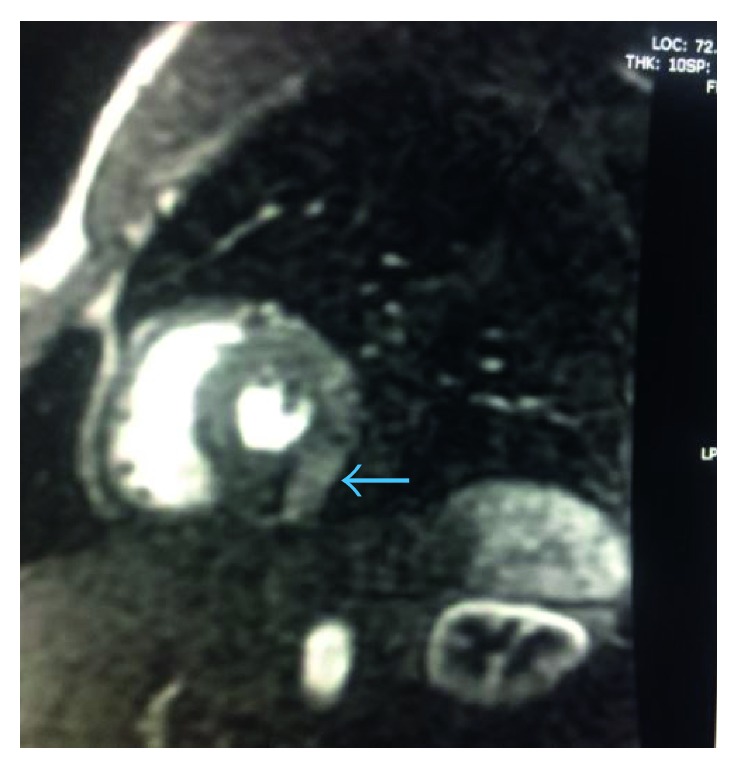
T1-weighted cardiac MRI short axis view demonstrating early enhancement ratios in the posterior epicardium and myocardium soon after gadolinium injection with sparing of the subendocardium (blue arrow).
